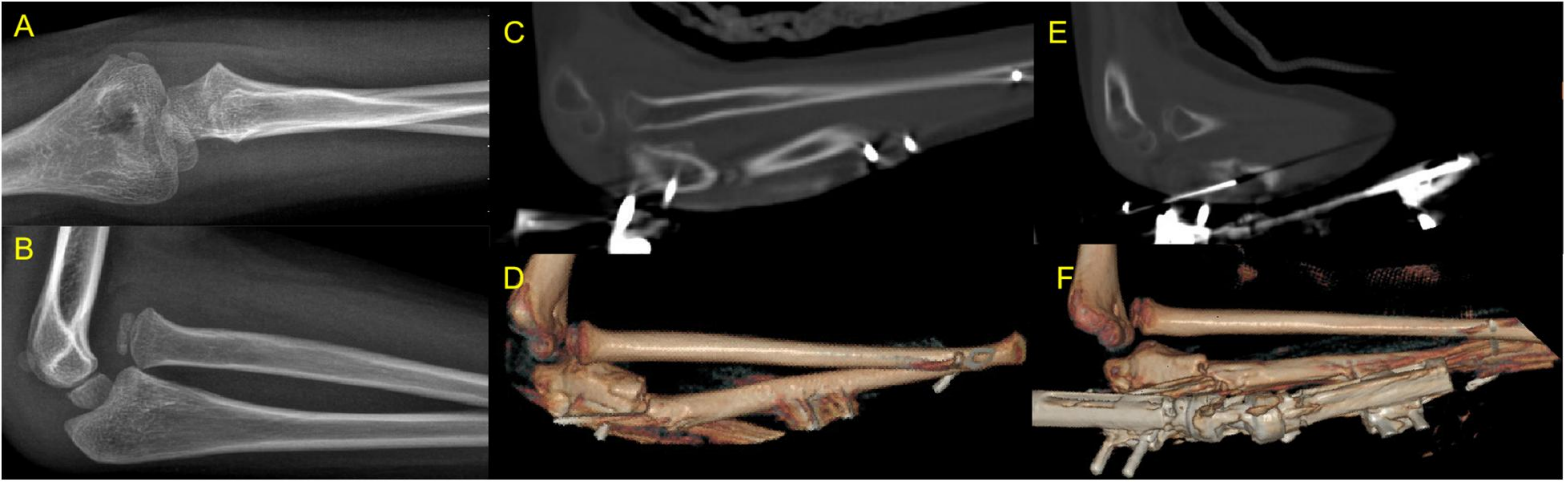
A surgical failure case with postoperative radial head redislocation. A 7-year-old male with elbow deformity 2 years after injury, showing radial head redislocation 1 month postoperatively. (A,B) Preoperative X-rays. (C,D) CT scan 2 days postoperatively showing reduced radial head. (E,F) CT scan 1 month postoperatively showing redislocation.
